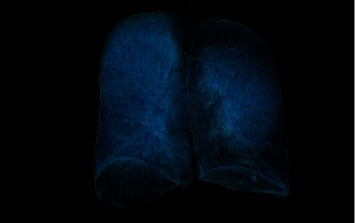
CT chest 3D reconstruction showing decreased lung field on bilaterally lower lobes and the left upper lobe.
